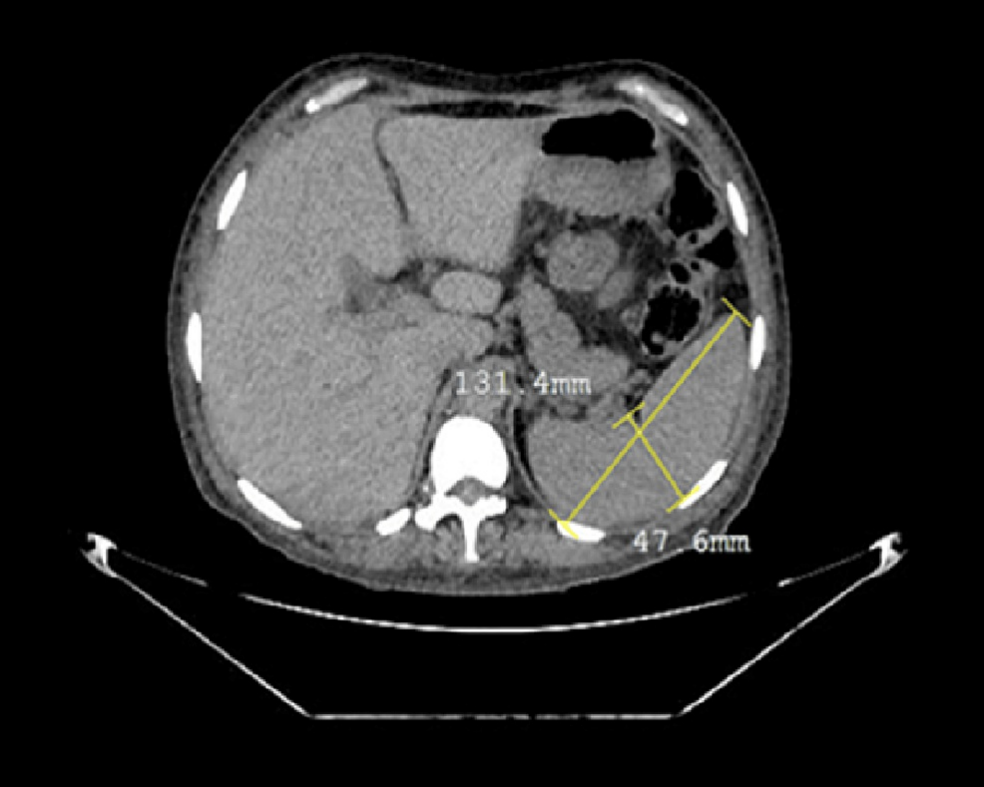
Abdominopelvic CT indicating splenomegaly The dimensions of the patient's spleen were 14.17 x 13.14 x 4.76 cm, and the splenic index measured 886.3 cm^3 ^(a value >440 cm^3^ suggests splenomegaly [4] CT: computed tomography
